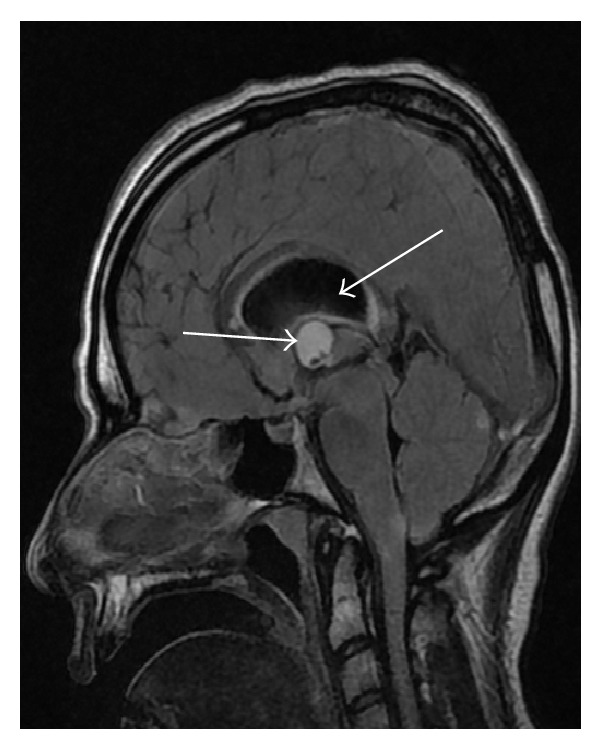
MRI brain of the same patient, sagittal view showing oval mass in the third ventricle at foramen of Monro, with hydrocephalus shown by arrows.
